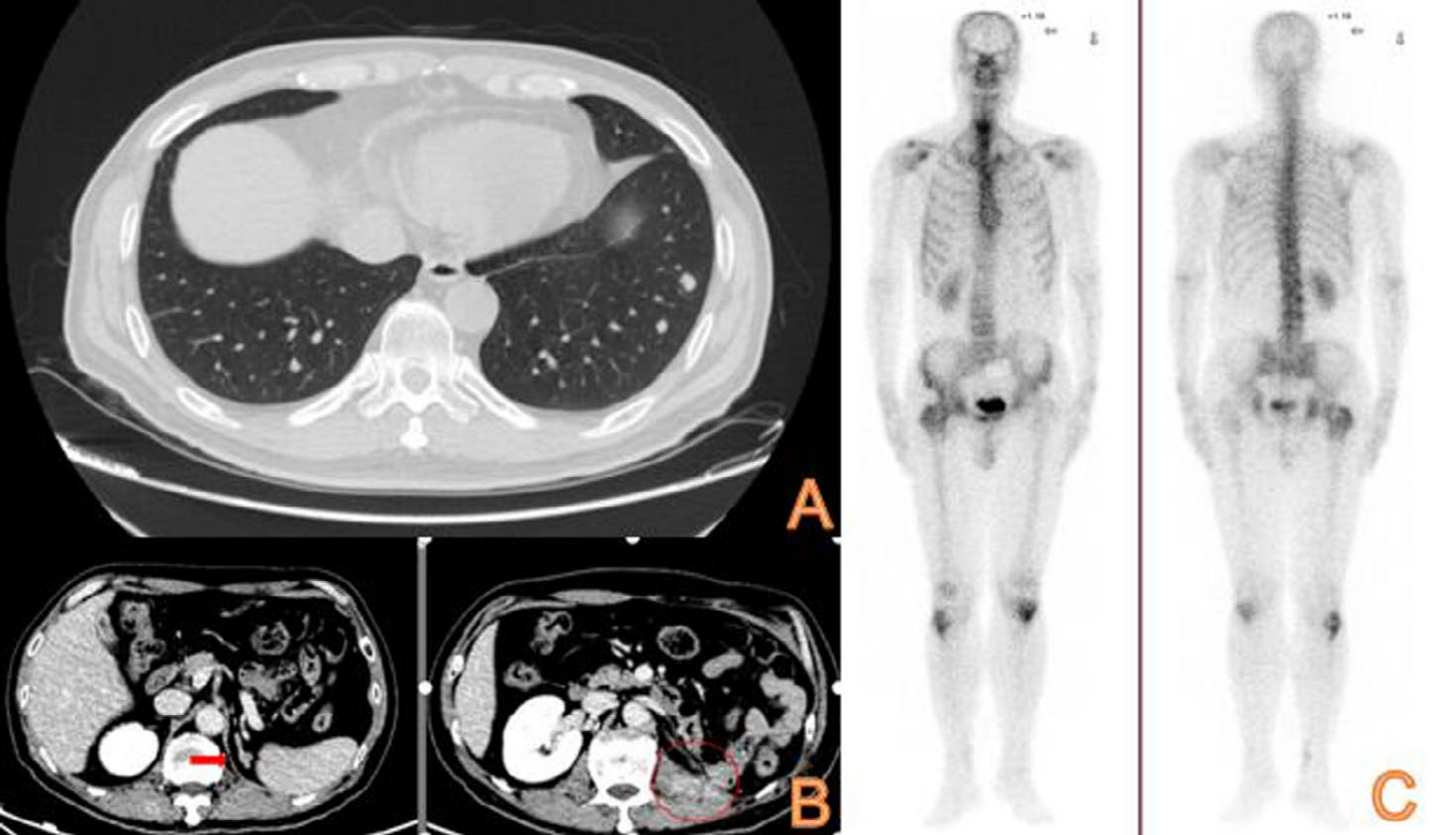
(A) Chest CT reveals lung metastases in May 2016. (B) Urinary CT reveals left adrenal metastasis in October 2017. (C) whole‐body radionuclide bone scan reveals metastatic lesions in the right acetabulum and upper femur in October 2016.
